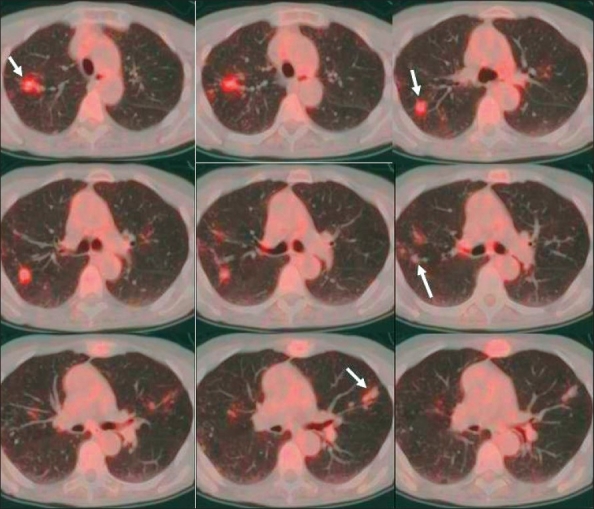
Fused axial, lung window, PET/CT images of the chest from a PET study of a 73-year-old patient with bilateral lung nodules. These generally show mild-to-moderate uptake (arrows), with more intense uptake in one of the right upper lobe nodules and with almost absent uptake in some of the left upper lobe nodules. Transbronchial biopsy was negative for malignancy and the patient was subsequently found to have Mycobacterium Avium-Complex (MAC) infection
